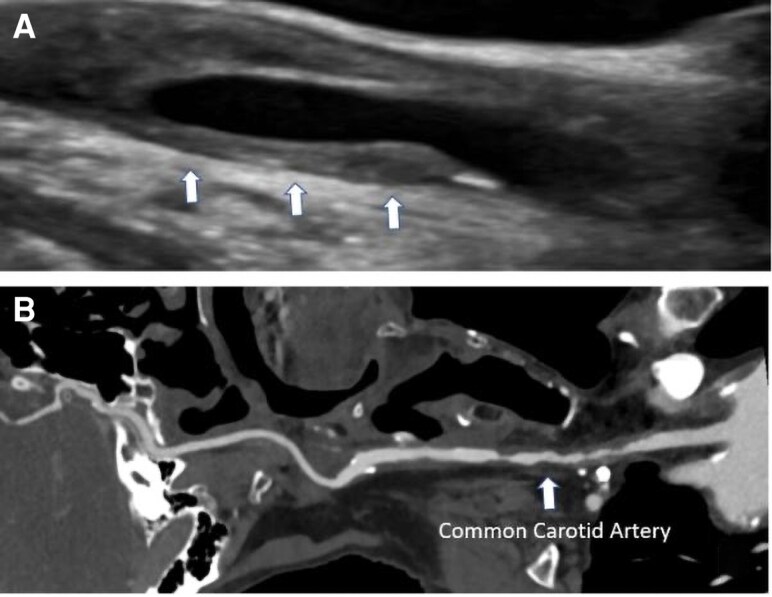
A 69-year-old male with a history of neck cancer, previously treated with surgical resection and neck radiotherapy, presented 13 years later for the consideration of coronary intervention. Ultrasound imaging (A) reveals diffuse non-calcified intimal-medial thickening (arrows) in the common carotid artery. Computed tomography angiography (B) shows diffuse non-calcified plaque disease in the common carotid artery, leading to multifocal severe stenosis.
